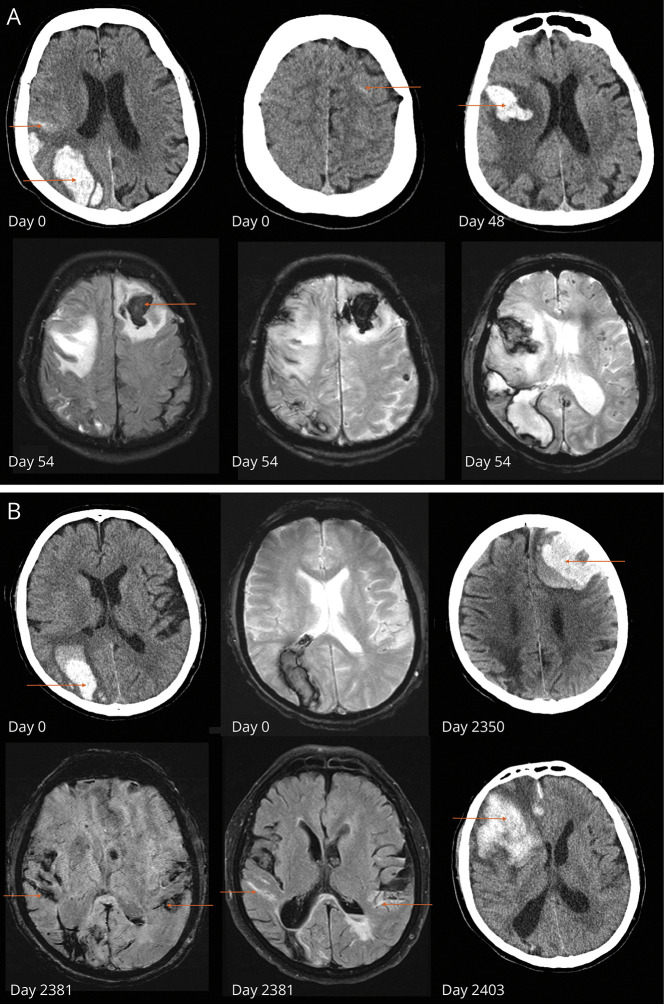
Neuroimaging Findings in 2 Exemplary Patients With ICH Clusters (A) A patient in their seventies with index right parietal intracerebral hemorrhage. On index CT, 2 additional separate foci of convexity subarachnoid hemorrhage were visible (right parietal, left frontal). 48 days after the index ICH, the patient suffered a recurrent ICH in the right frontal lobe. On MRI after clinical worsening 6 days later, an additional large left frontal ICH was visible, as were disseminated cortical superficial siderosis and numerous lobar microbleeds. This patient did not have any further recurrent ICH over a follow-up period of 9 years (with several CT scans performed in the follow-up period). (B) Another patient in their seventies with right occipital intracerebral hemorrhage. T2* MRI sequences did not show additional chronic hemorrhagic lesions. 6 years later, the patient suffered a left frontal hemorrhage. After initial stabilization, MRI depicted bilateral parietal acute convexity subarachnoid hemorrhage and extensive cortical superficial siderosis. 53 days after the second ICH, the patient had a large right frontal ICH and died shortly thereafter. ICH = intracerebral hemorrhage.
